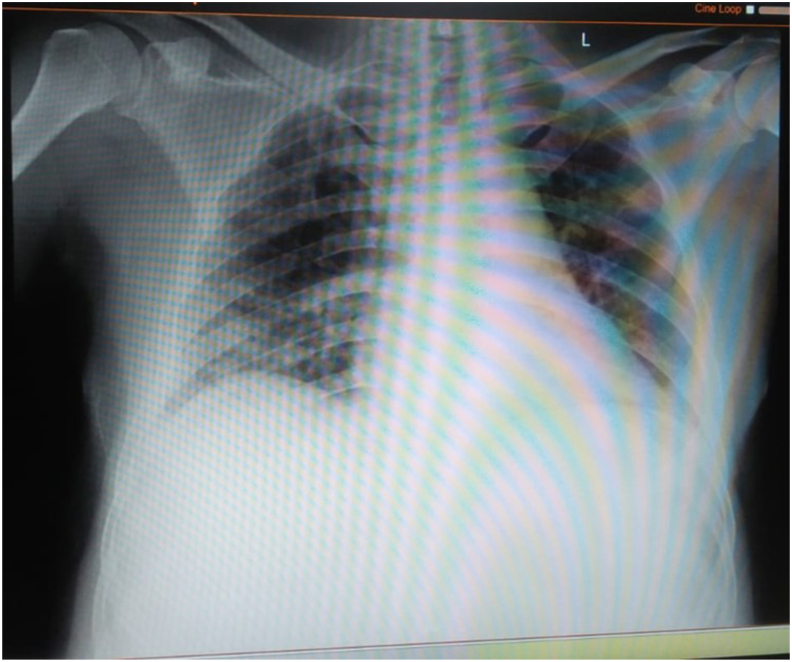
Chest X-ray revealed inhomogeneous opacity on bilateral paracardial and lateral aspects.
